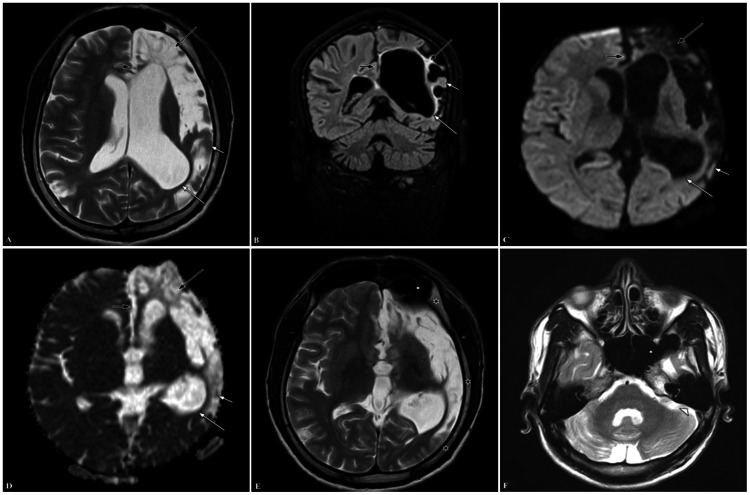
Case presentation 1 - MRI brain T2W axial (A), FLAIR coronal (B), DWI axial (C), and ADC axial (D) show left hemicerebral atrophy (short white arrow). There is a hyperintense signal on T2W, a hypointense signal on FLAIR, and a corresponding hypointense signal on DWI and a hyperintense signal on ADC in the cortical and subcortical white matter of the left cerebral hemisphere (predominantly in the left frontal lobe). A thin rim of a hyperintense signal on FLAIR representing gliosis (long black arrow) causing ipsilateral lateral ventricle ex vacuo dilatation (long white arrow) is indicated. There is a minimal midline shift of falx cerebri to the ipsilateral side (short black arrow). T2W axial (E, F) show compensatory ipsilateral calvarial hypertrophy (black asterisks), ipsilateral frontal and sphenoid sinus hyperpneumatization (white asterisks), and ipsilateral mastoid air cell hyperpneumatization (small white arrowhead), respectively. MRI: magnetic resonance imaging; T2W: T2-weighted; FLAIR: fluid attenuation inversion recovery; DWI: diffusion-weighted imaging; ADC: apparent diffusion coefficient
